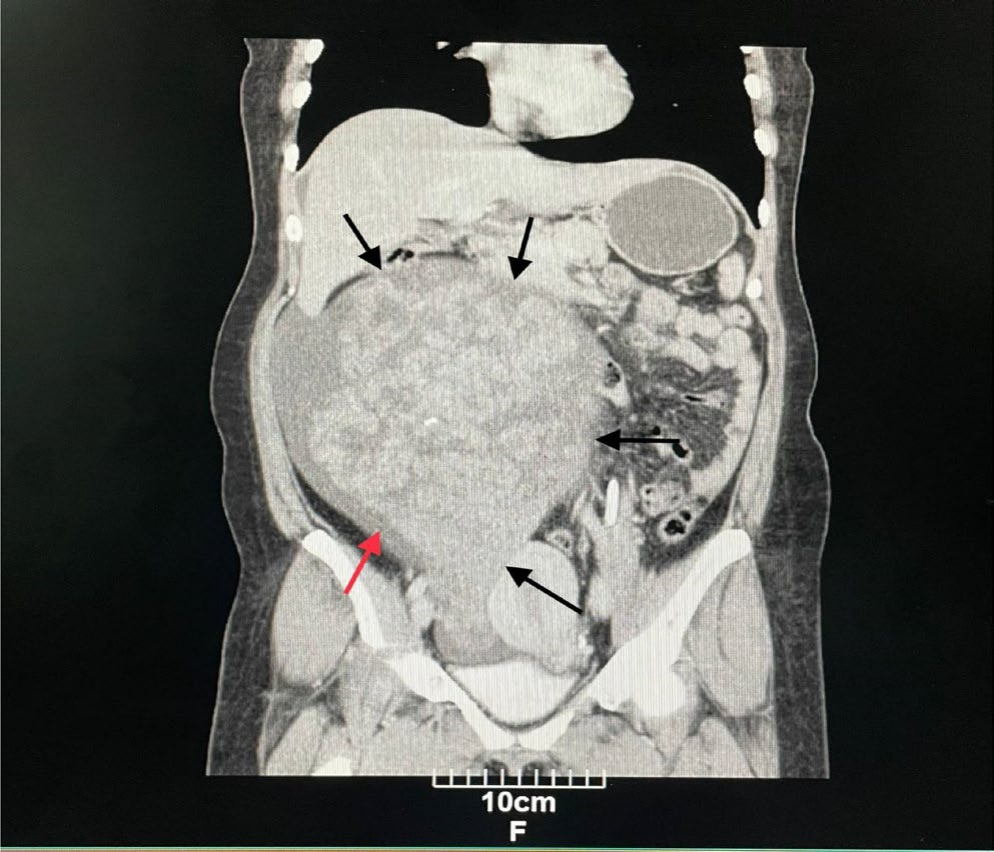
CT scan of the abdomen and pelvis (coronal view) showing huge pelvi‐abdominal mass compressing the intestinal loops and uterus (black arrows) also showing ascites fluid (red arrow).
